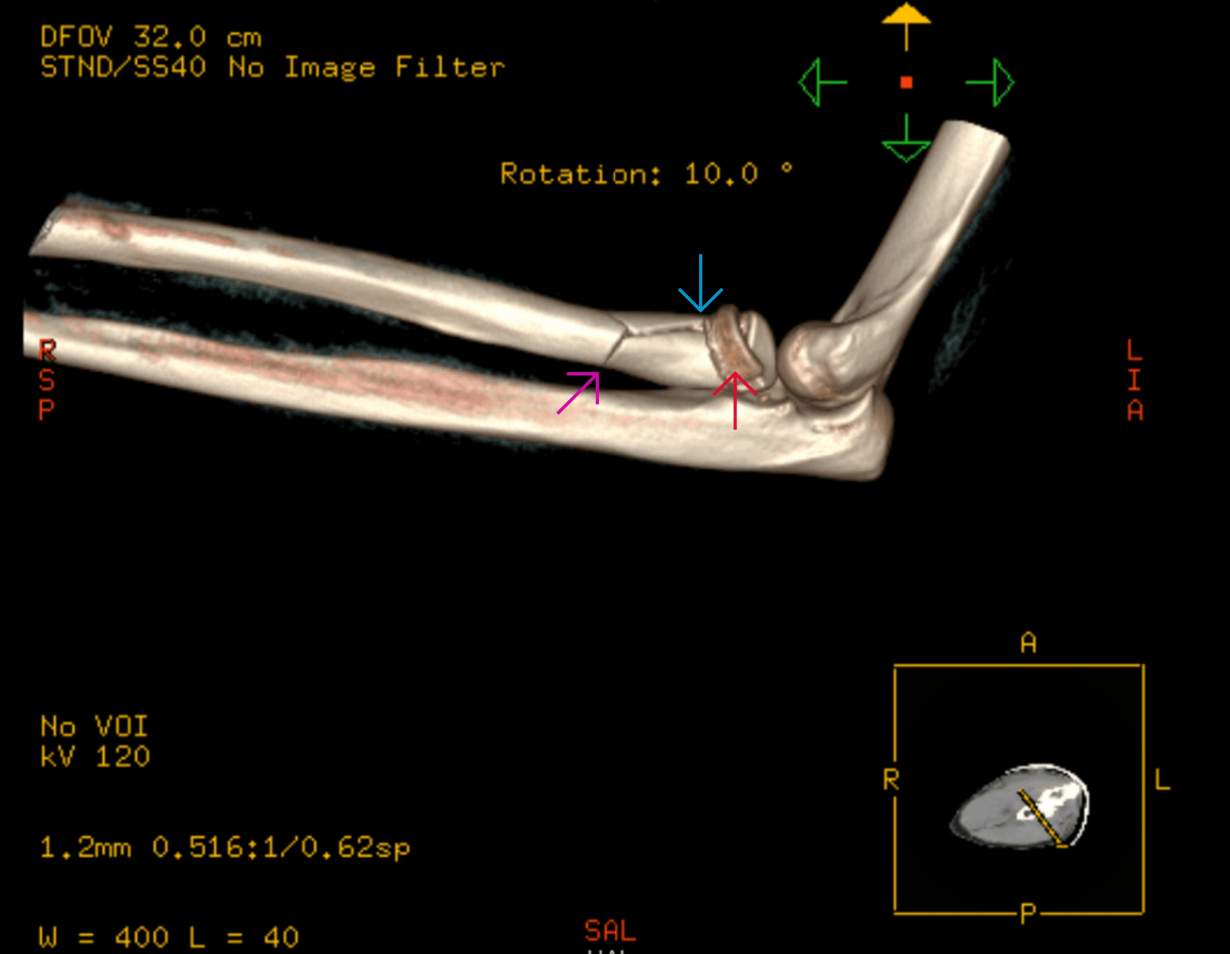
CT scan image showing the radial head (red arrow), neck (blue arrow), and shaft (pink arrow) fracture with intact ulna
